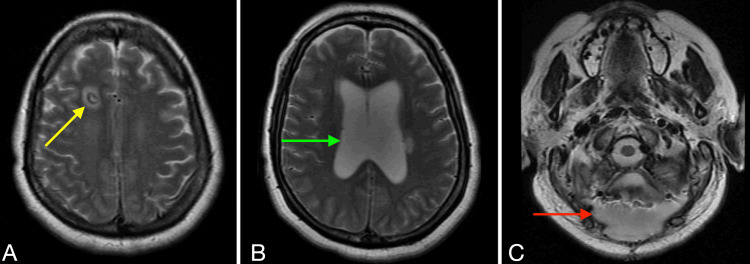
Axial 2D T2-weighted brain MRI analysis. (A) Frontal extraventricular drain: The image shows moderate enhancement along the drain tract, indicated by the tip of the yellow arrow. (B) Hydrocephalus: Moderate hydrocephalus is visible, located at the tip of the green arrow. (C) Suboccipital craniotomy postoperative changes: The image displays postoperative changes within the fourth ventricle, showing an accumulation of fluid external to the cranial vault, which indicates CSF leakage. This is found at the tip of the red arrow.
